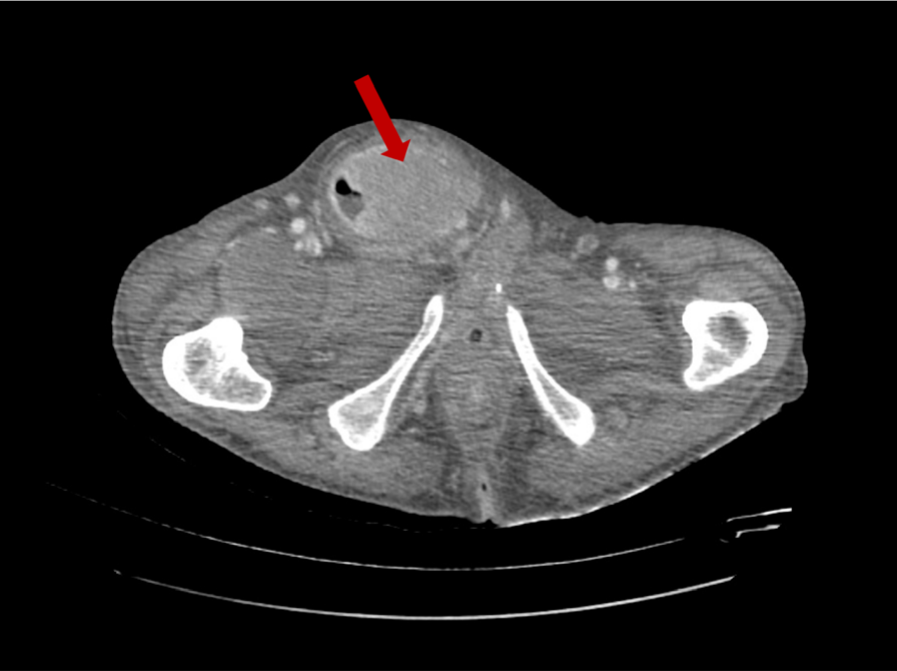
CT scan. The ileal loop located in the inguinal canal judged by the radiologist to be fixed and non-reducible
